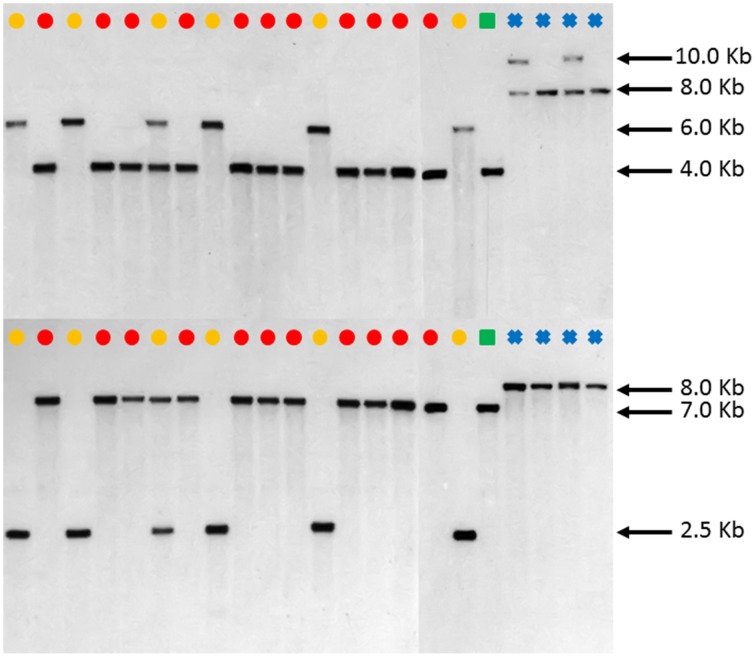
Southern blot *FAD2-1* digestion radiographs (top using enzyme *Bgl*II, bottom using enzyme *Eco*RV). Samples are in order according to their listing in Table 1 starting with PI 209295 and ending with PI 426488.
